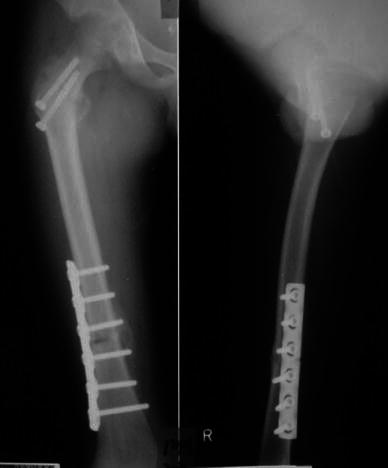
At six weeks after the operation, the radiographs show well-maintained reduction. The patient was started on non-weight-bearing active exercises
